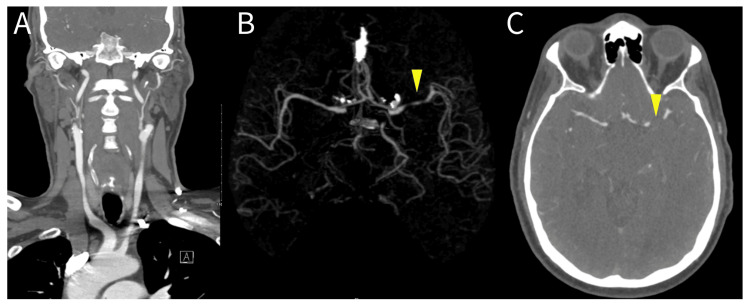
CT Angiogram of the head and neck: A) Atheromatous changes in the internal carotid arteries without associated stenosis; B & C) 3D reconstruction of CT Angiogram and axial image of CT Angiogram of the brain. Yellow arrowheads point towards the patient's left MCA stenosis. MCA: middle cerebral artery
